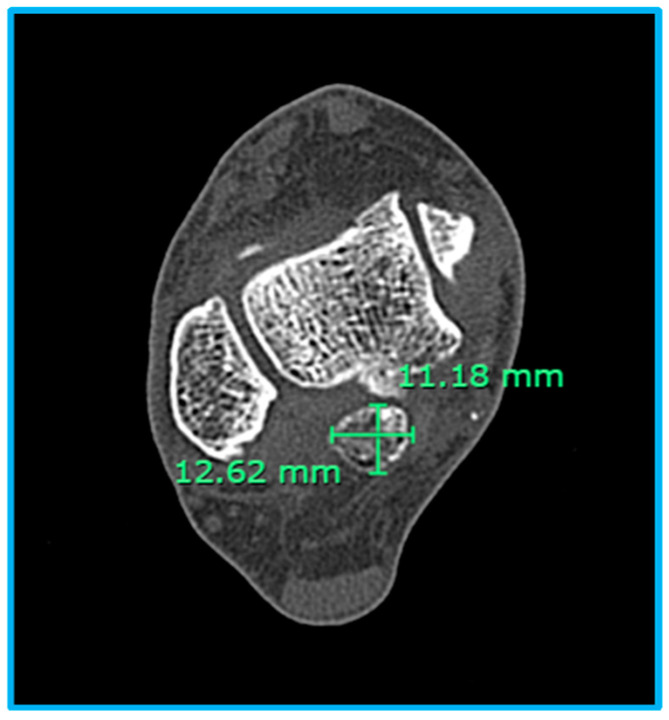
A CT axial view of the ankle demonstrates a Type I os trigonum. The os trigonum is measured, revealing a macro-axis of 12.62 mm and a minor-axis of 11.18 mm.
